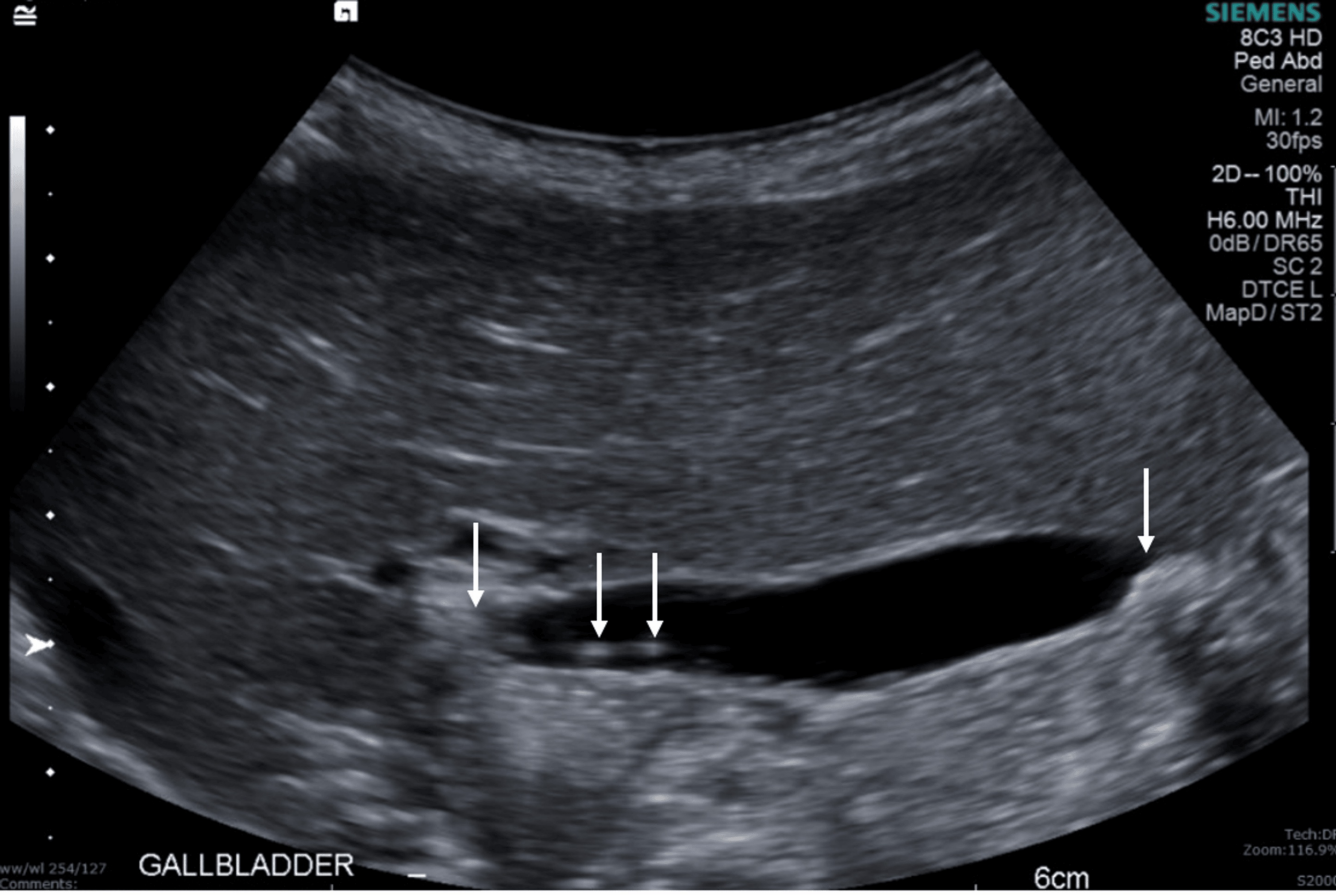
Follow-up abdominal ultrasound Follow-up abdominal ultrasound (gallbladder view) showing interval resolution of previously seen intra- and extrahepatic biliary duct dilatation and cholelithiasis without cholecystitis, including a 6 mm gallstone in the neck of the gallbladder.
